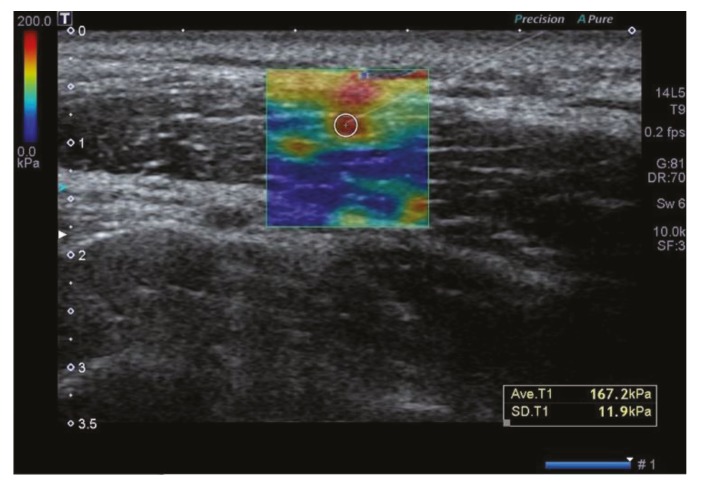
Ultrasound-guided myofascial trigger point (MTrP) injection. Increased stiffness at the MTrPs on shear wave elastography is confirmed on shear wave elastography. The injection was followed at the point where the stiffness increased mostly in the region of interest.
